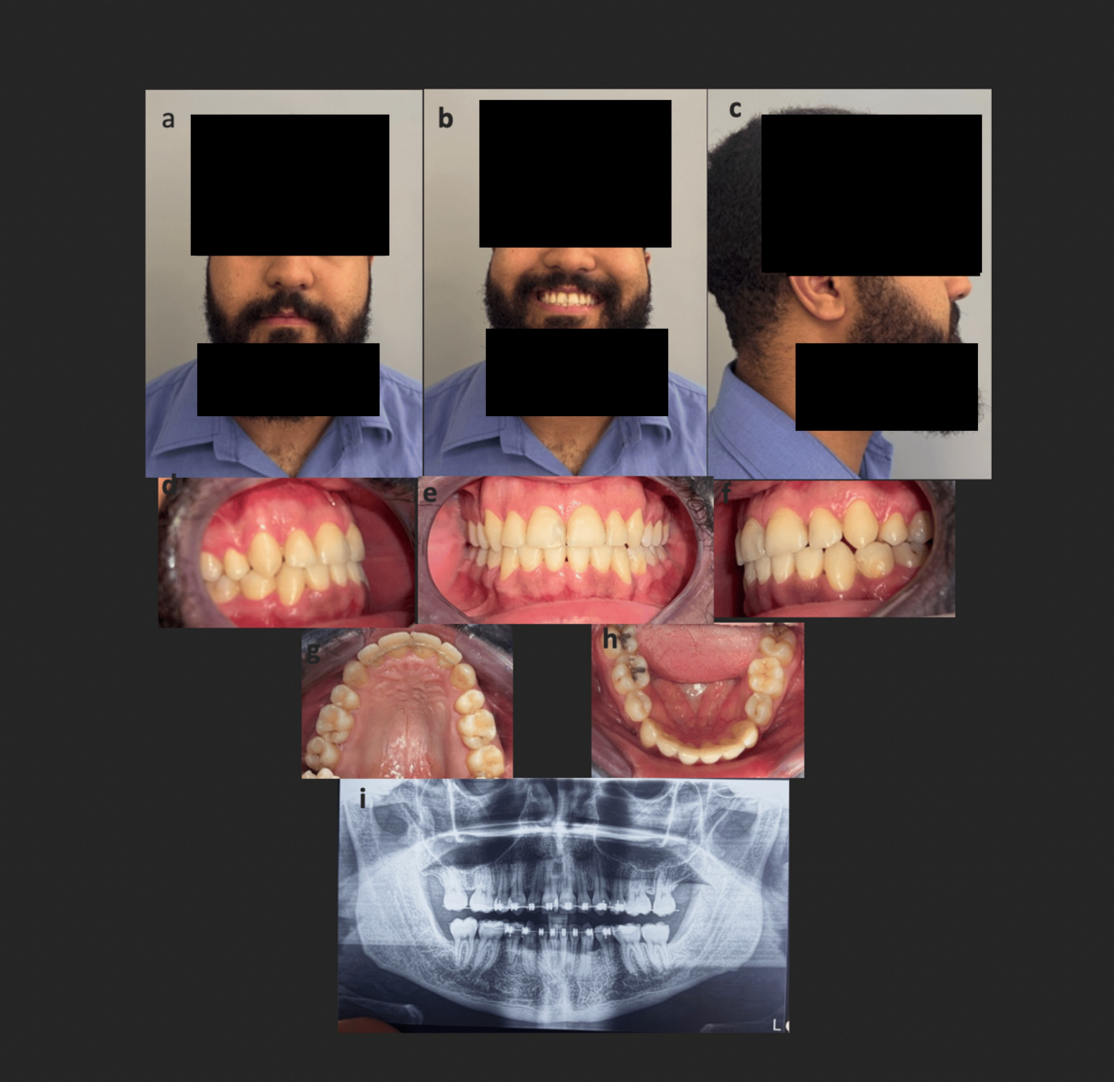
Extraoral (a, b, c) and intraoral (d, e, f, g, h) images and panoramic radiograph (i) showing clinical outcomes after 20 months of treatment. The extraction of the wisdoms was carried out given their unfavorable axes for the eruption.
